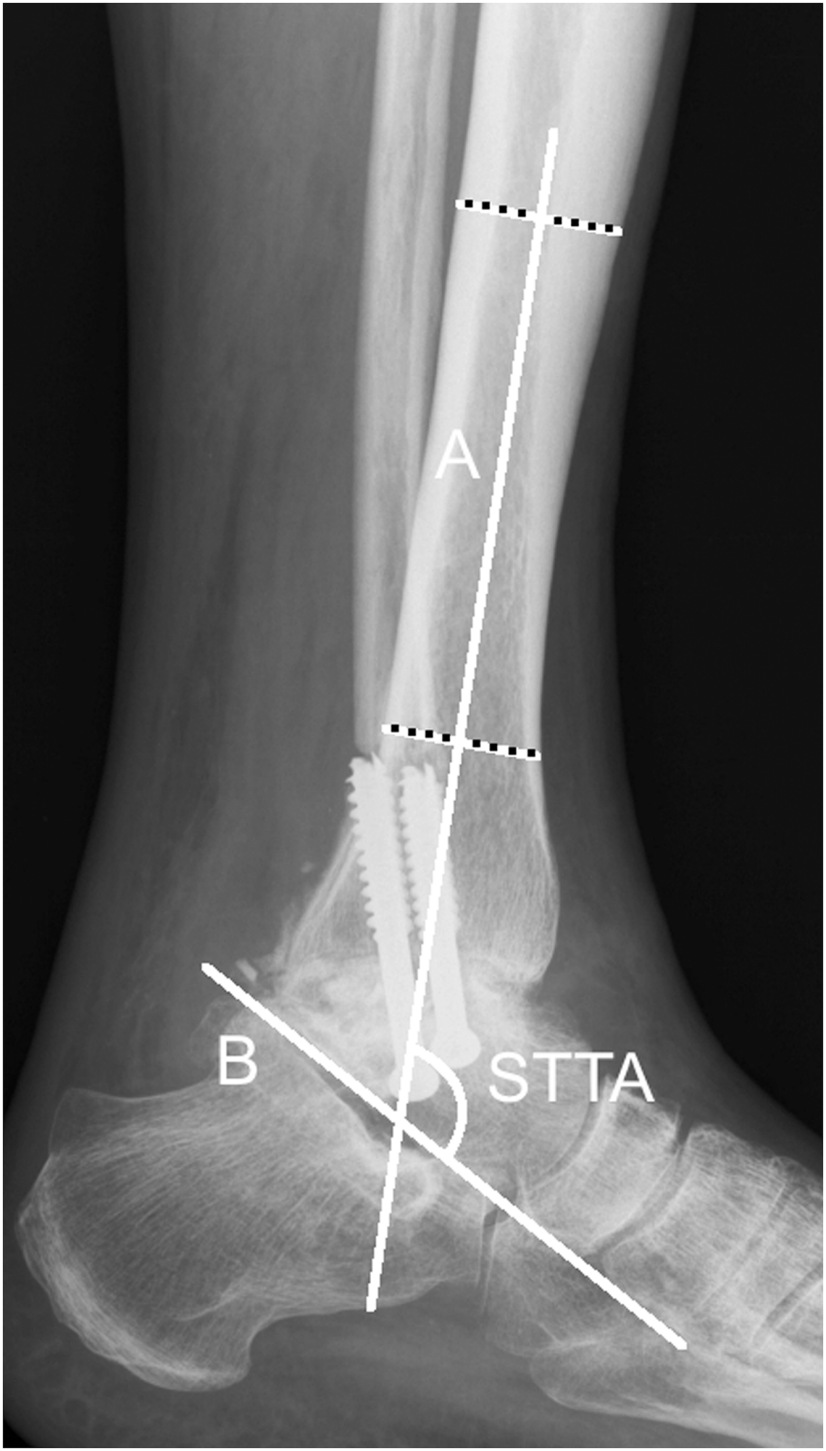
Lateral radiograph ankle arthrodesis. Lateral ankle radiograph with the measurement of the sagittal tibiotalar angle (STTA). The STTA is the angle between the the two axes A and B. A = longitudinal axis of the tibia, created by connecting two points in the middle of the proximal and the distal tibial shaft (according to method B). B = axis of the talus, defined by a line drawn from the inferior aspect of the posterior tubercule of the talus to the most inferior aspect of the talar neck.
